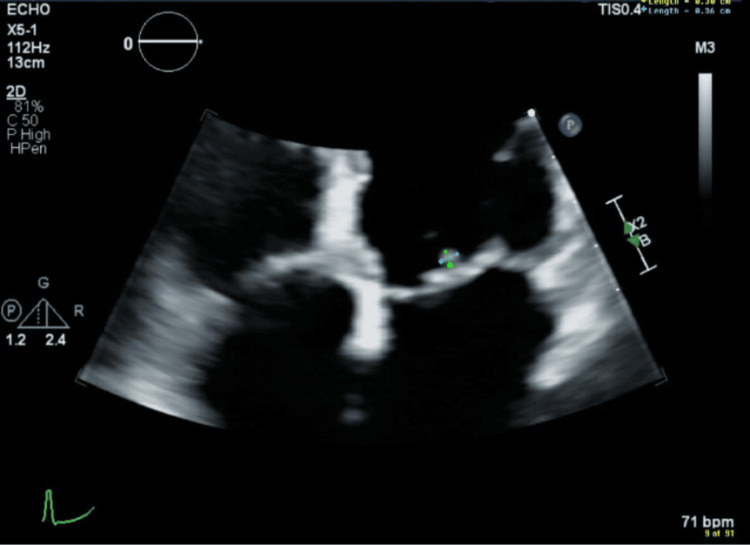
Echocardiogram Echocardiogram image showing questionable early vegetation (outlined in green) versus mild thickening of mitral valve leaflets.
